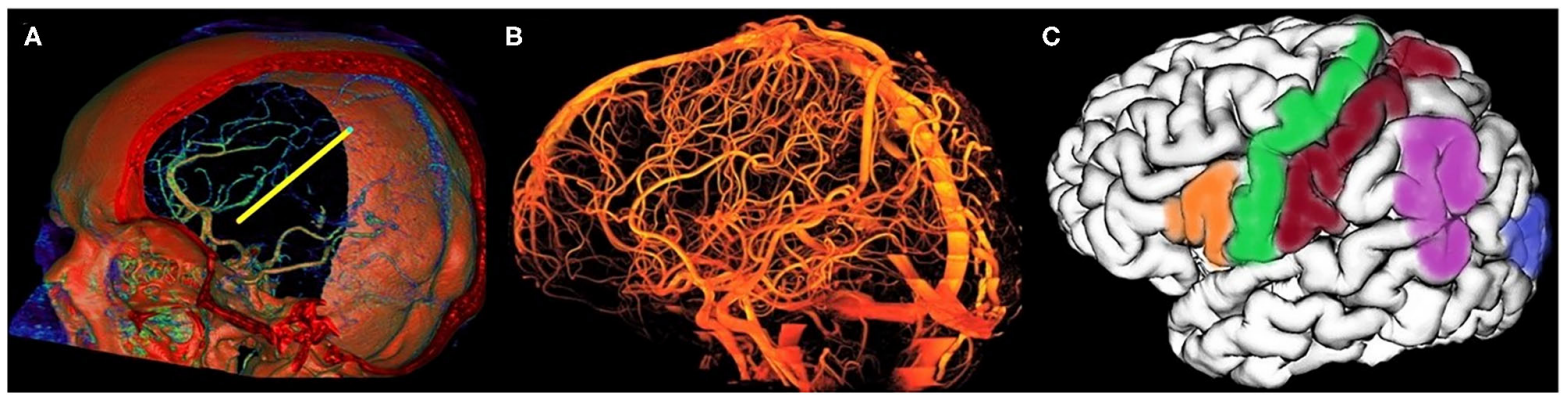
Avoiding all visible vessels is critical in stereotactic planning. (A) A 3D reconstruction of human cerebral arterial system with a planned trajectory depicted in yellow—the planned trajectory is shown from the stereotactic planning module RONNAplan (Jerbić et al., 2020); (B) a 3D reconstruction of a time of flight magnetic resonance angiography showing a complete vascular system of the human brain; (C) a 3D MRI reconstruction of left cerebral hemisphere with eloquent brain areas marked in colors (green—motor area, red—sensory area, blue—visual area, orange—Broca's area, and purple—Wernicke's area).
